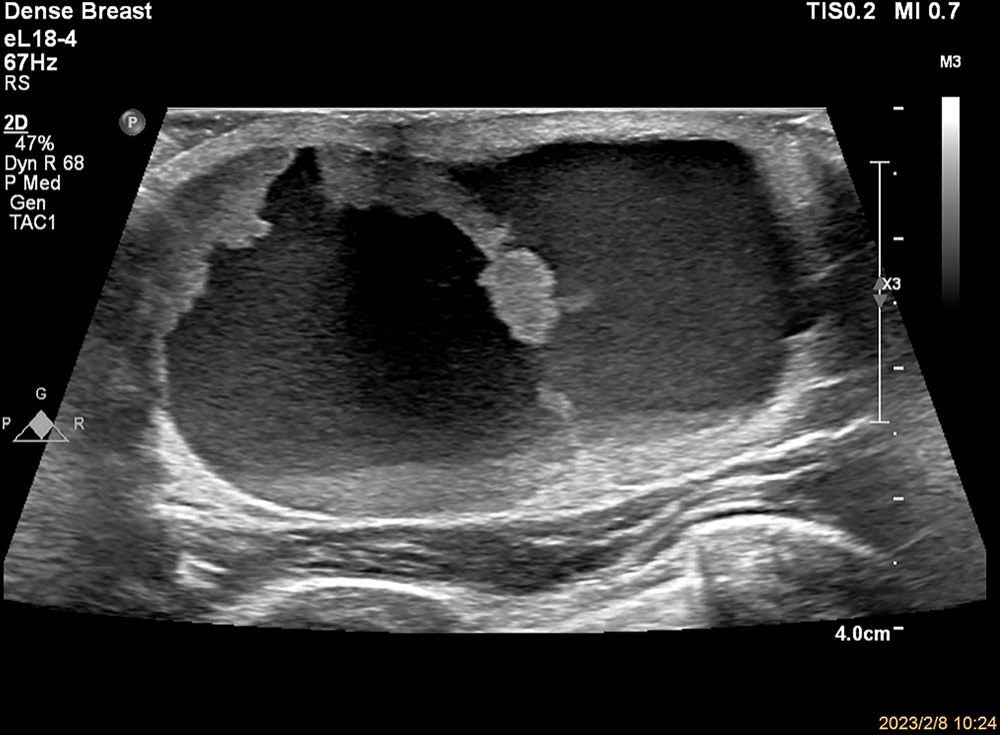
Male, 61 years old, intraductal papilloma of the breast, gray-scale ultrasound showing subcutaneous mixed echogenic mass.
